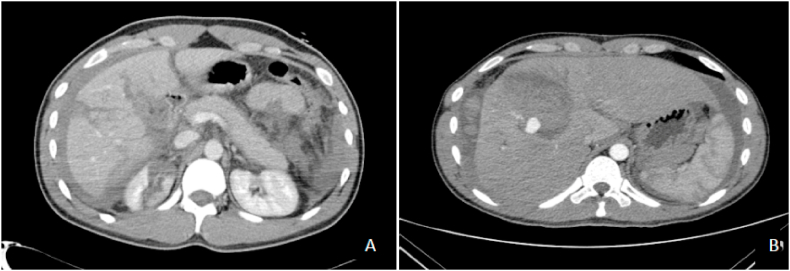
CT scan findings showing hepatic capsular and parenchymal laceration affecting segments V, VI and VIII (A) with subcapsular hematoma (B) formation at posterior aspect of right liver lobe.
